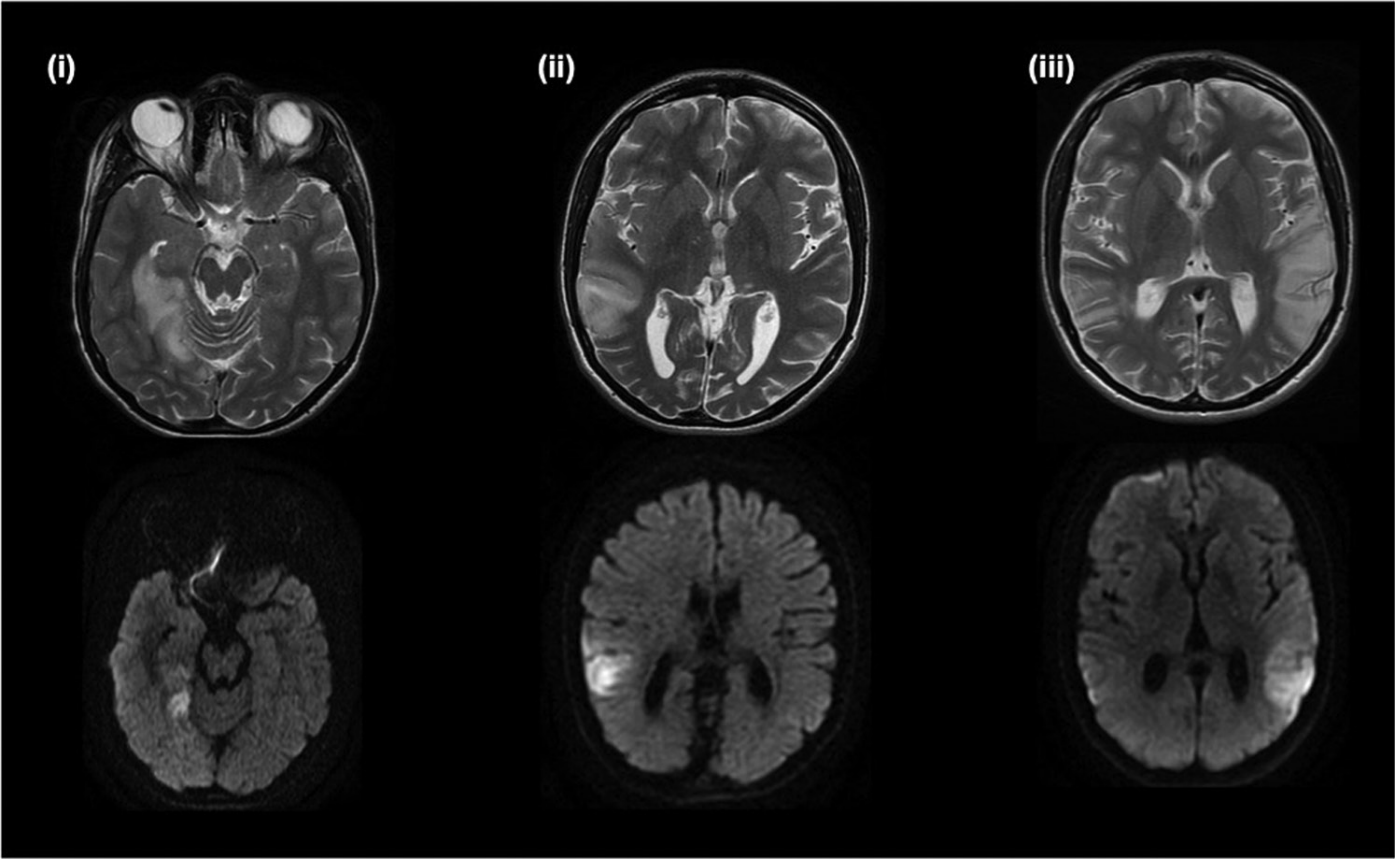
Brain MRIs, axial view T2‐weighted (first row) and DWI (second row), showing development and resolution of signal abnormalities within the (i) right medial temporal lobe upon initial presentation, (ii) right lateral temporal lobe 4 months after initial presentation, and (iii) left lateral temporal lobe 5 months after initial presentation
